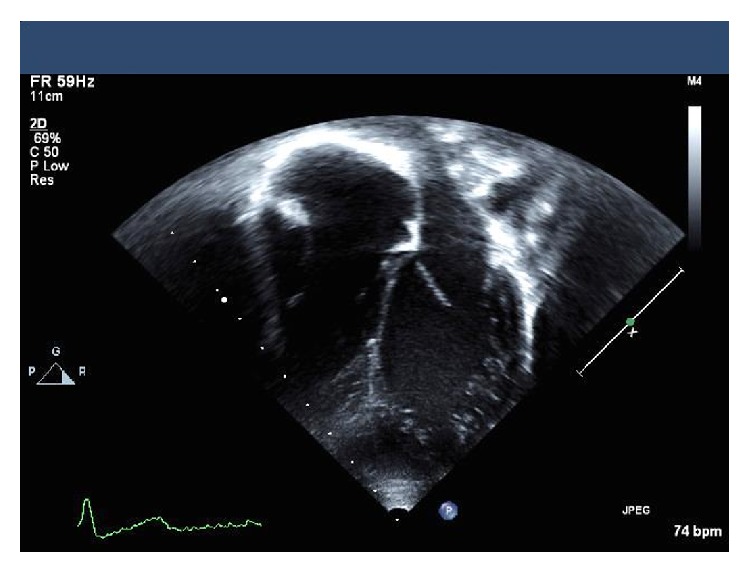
Echocardiography obtained from the apical 4-chamber view demonstrating apical trabeculation consistent with left ventricular noncompaction.
